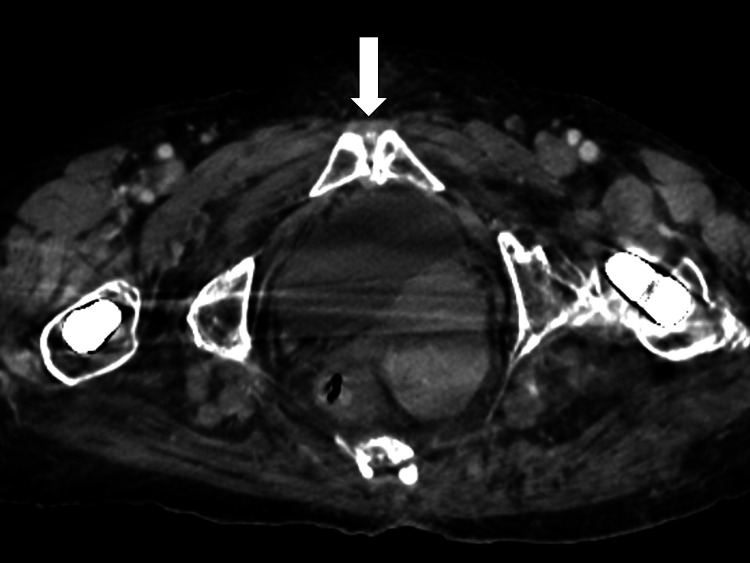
A chest-to-pelvic computed tomography image revealing calcification around the shoulder joint and the pubic symphysis (indicated by white arrows).
